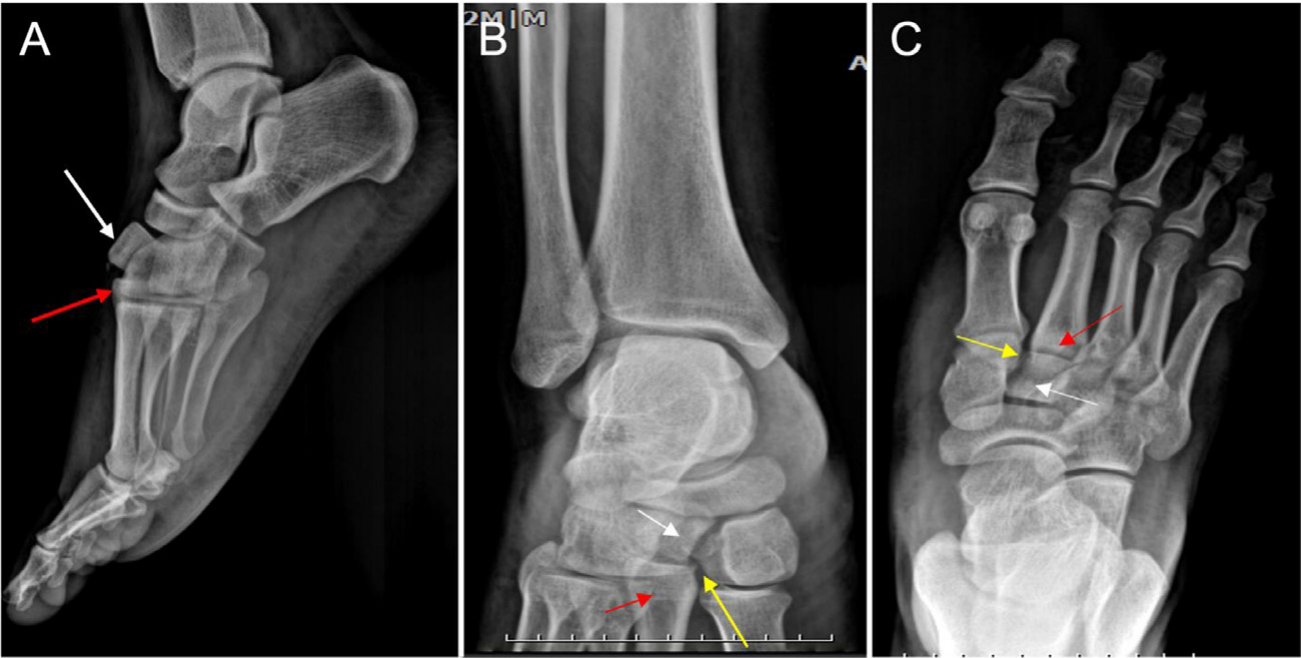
Plain radiographs of the right foot taken prior to surgery. (A) Lateral (mediolateral) view, (B) Oblique view, and (C) Anteroposterior (AP) view. The images clearly show a Lisfranc-type injury with dorsal dislocation of the intermediate cuneiform (white arrows) and second metatarsal (red arrows). Disruption is also seen between the medial cuneiform and the second metatarsal base, known as the “fleck sign” (yellow arrows).
